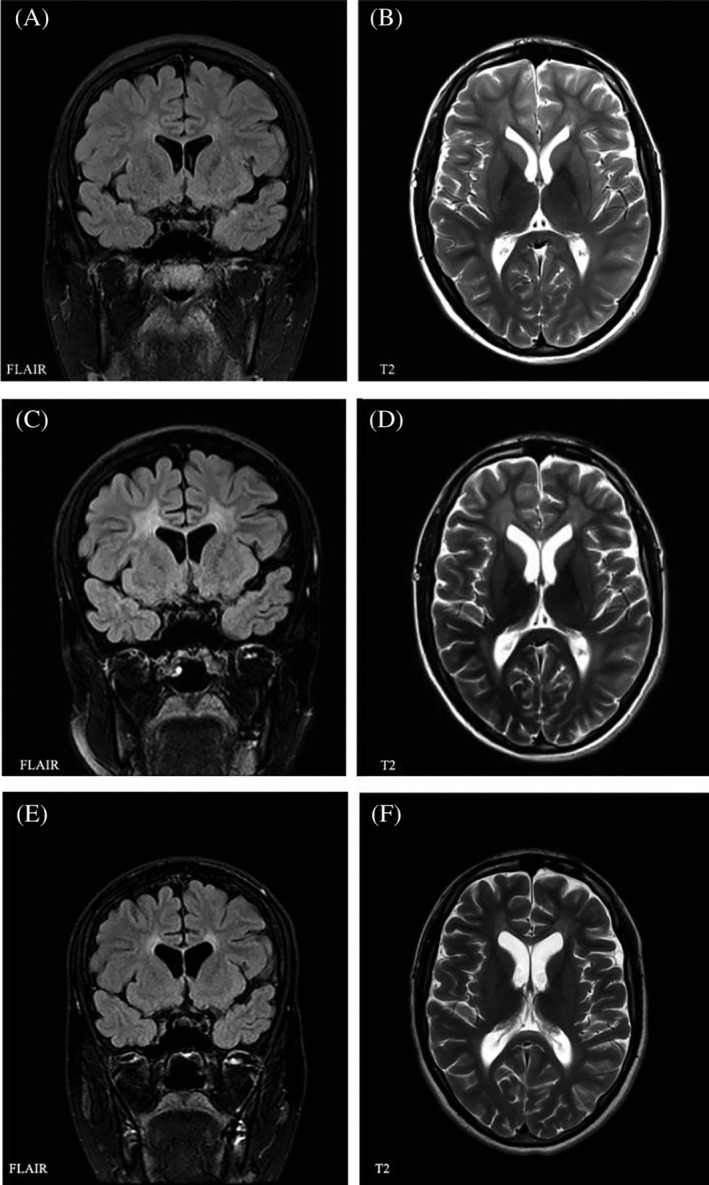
Brain MRI patient B: Brain MRI of patient B before HSCT and 9 and 37 months after. A (Coronal section, FLAIR) and B (Axial section, T2 weighting) were performed one month before transplantation (age 18 years) showing mild white matter pathology in the frontal and parieto‐occipital region as well as the periventricular and central region corresponding to an MLD‐MRI score of 5/34. C (Coronal section, FLAIR) and D (Axial section, T2 weighting) were performed 9 months after transplantation (age 18 years and 10 months) showing symmetrical, periventricular white matter changes in the cerebral hemispheres as well as a slight involvement of U‐fibres in few gyri. The findings correspond to an MLD‐MRI score of 13/34. E (Coronal section, FLAIR) and F (Axial section, T2 weighting) were performed 37 months after transplantation (age 19 years, 9 months) showing a decreased distribution and intensity of white matter pathology, no longer with involvement of U‐fibres. The findings correspond to an MLD‐MRI score of 11/34
